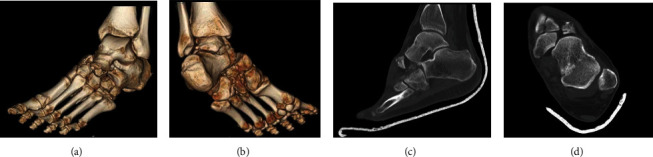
(a) 3D CT scan demonstrating swivel injury, comminuted fracture of the navicular and cuboid bone, and calcaneocuboid joint subluxation. (b) 3D CT scan from medial viewpoint showing the medial dislocation of the talonavicular joint. (c) Sagittal CT cut of the left foot showing both talonavicular and subtle calcaneocuboid joint subluxations. (d) Axial cut of the CT scan showing medial dislocation of the talonavicular joint left foot; note the navicular fracture fragment that remained in the anatomic position; this “constant fragment” received screw fixation to aid in reconstruction of the navicular bone.
